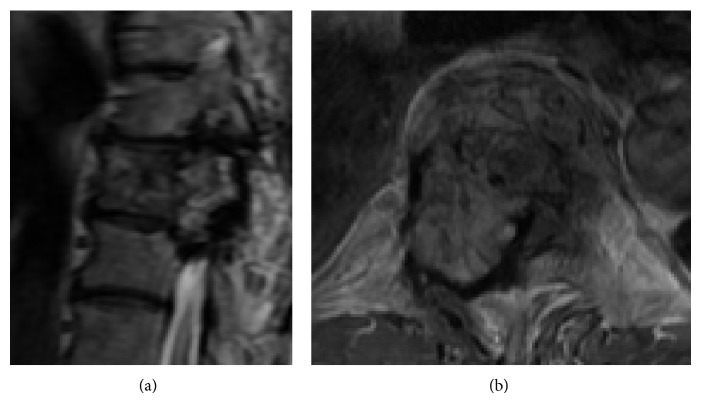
Preoperative MRI showing a multilevel invasive vertebral tumor with extraosseous extension. (a) Sagittal T2-WI, (b) axial gadolinium-DTPA-enhanced T1-WI image (T9).
